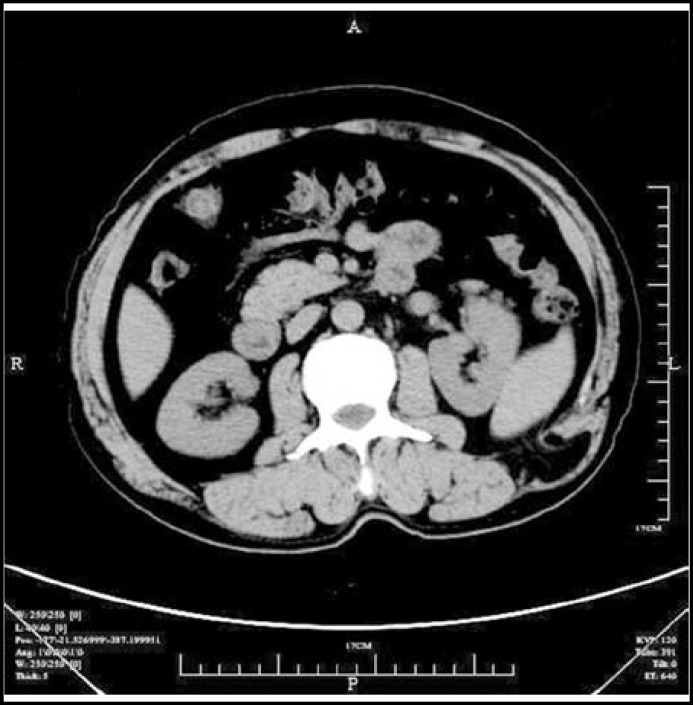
Computed tomography (CT) scan demonstrating left lumbar hernia containing fatty tissue
